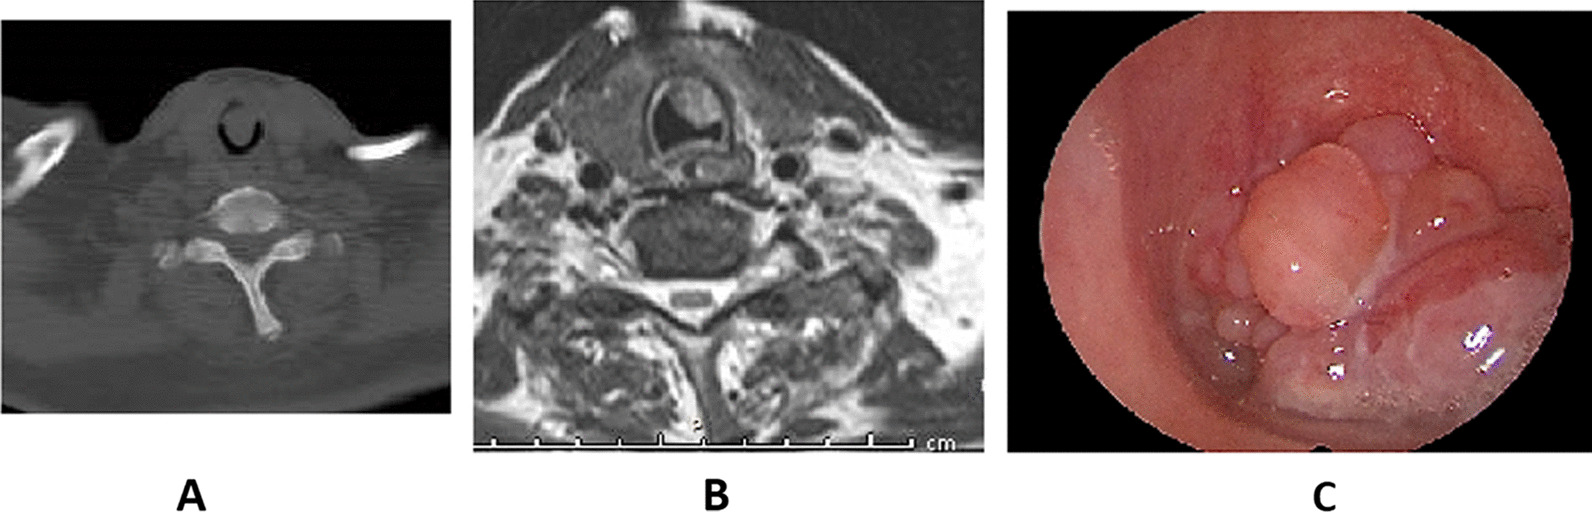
a CT scan showing the mass in the subglottic region and upper trachea. b MRI showing the mass without definite extra tracheal extension. c Direct laryngoscopy shows a lobulated mass in the upper trachea, occluding entire airway
